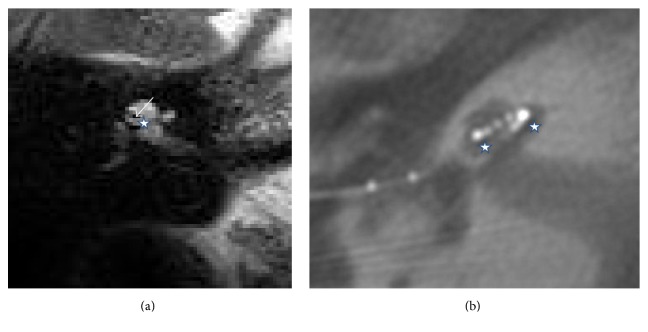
(a) MRI scalar pattern of the scala vestibuli's position in Patient 9. The star indicates the scala tympani in the second turn. The arrow indicates the electrode's diminishing signal in the scala vestibuli. (b) FPT pattern of the scala vestibuli's position in Patient 9. The stars indicate the scala tympani in the basal and second turns.
